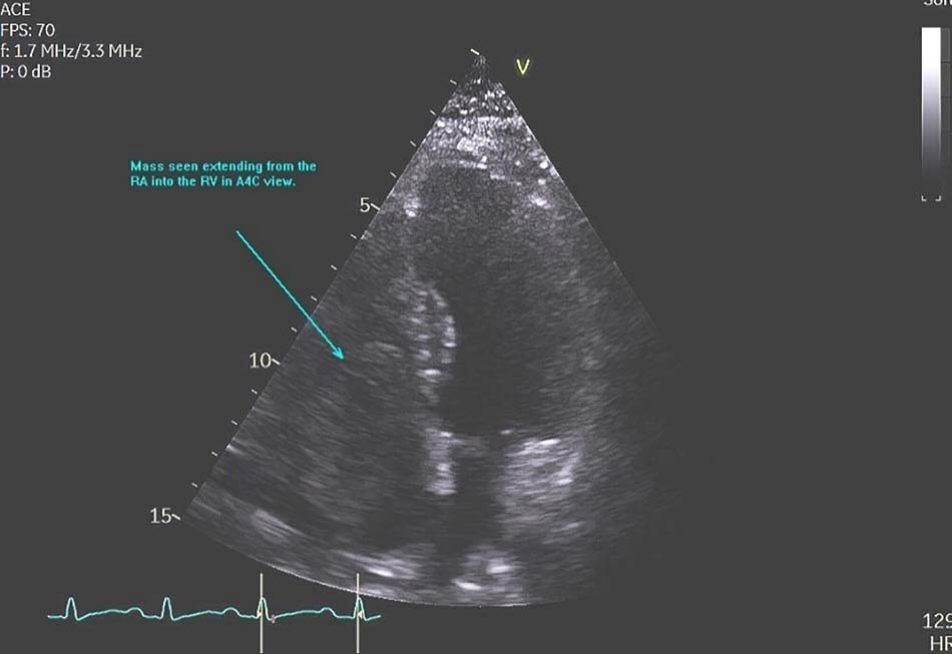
TTE showing RA myxoma seen extending into the RV in the apical four chamber view.
